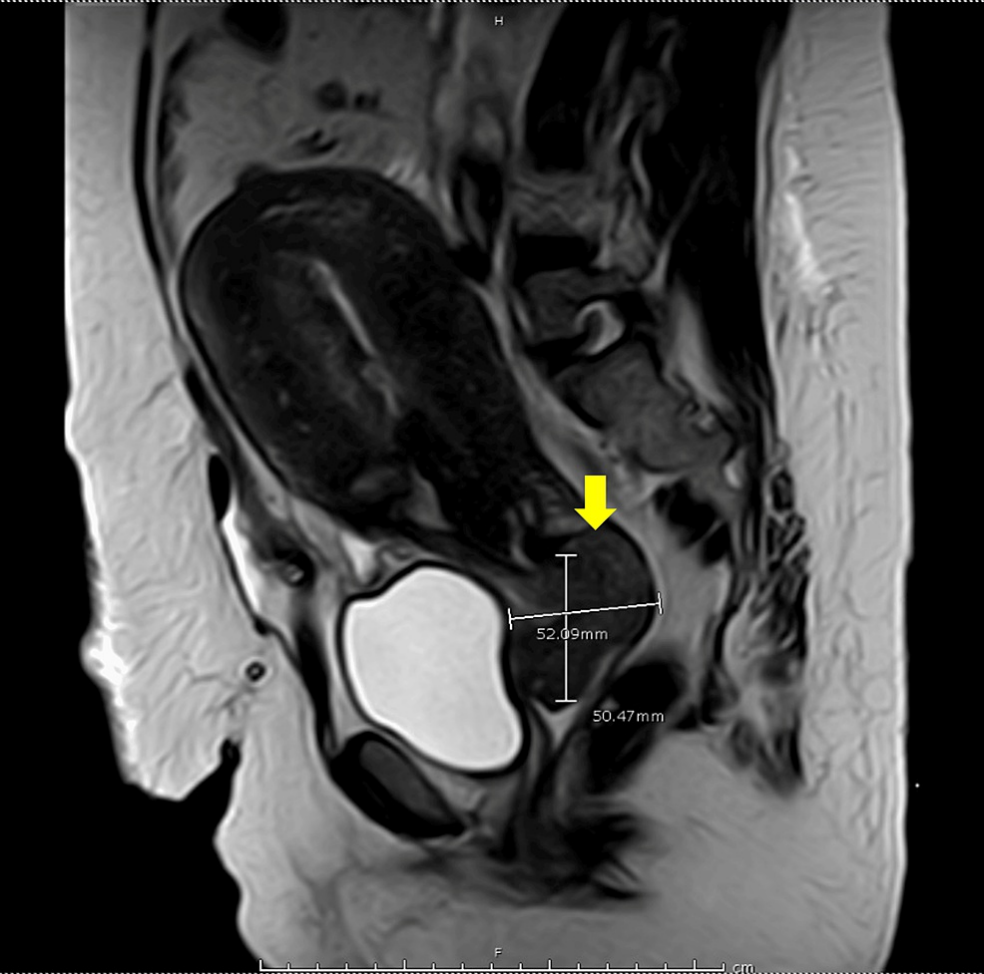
Abdominopelvic T2-weighted MRI showing enhanced soft tissue cervical mass measuring 5.2x5 cm. MRI: magnetic resonance imaging.
